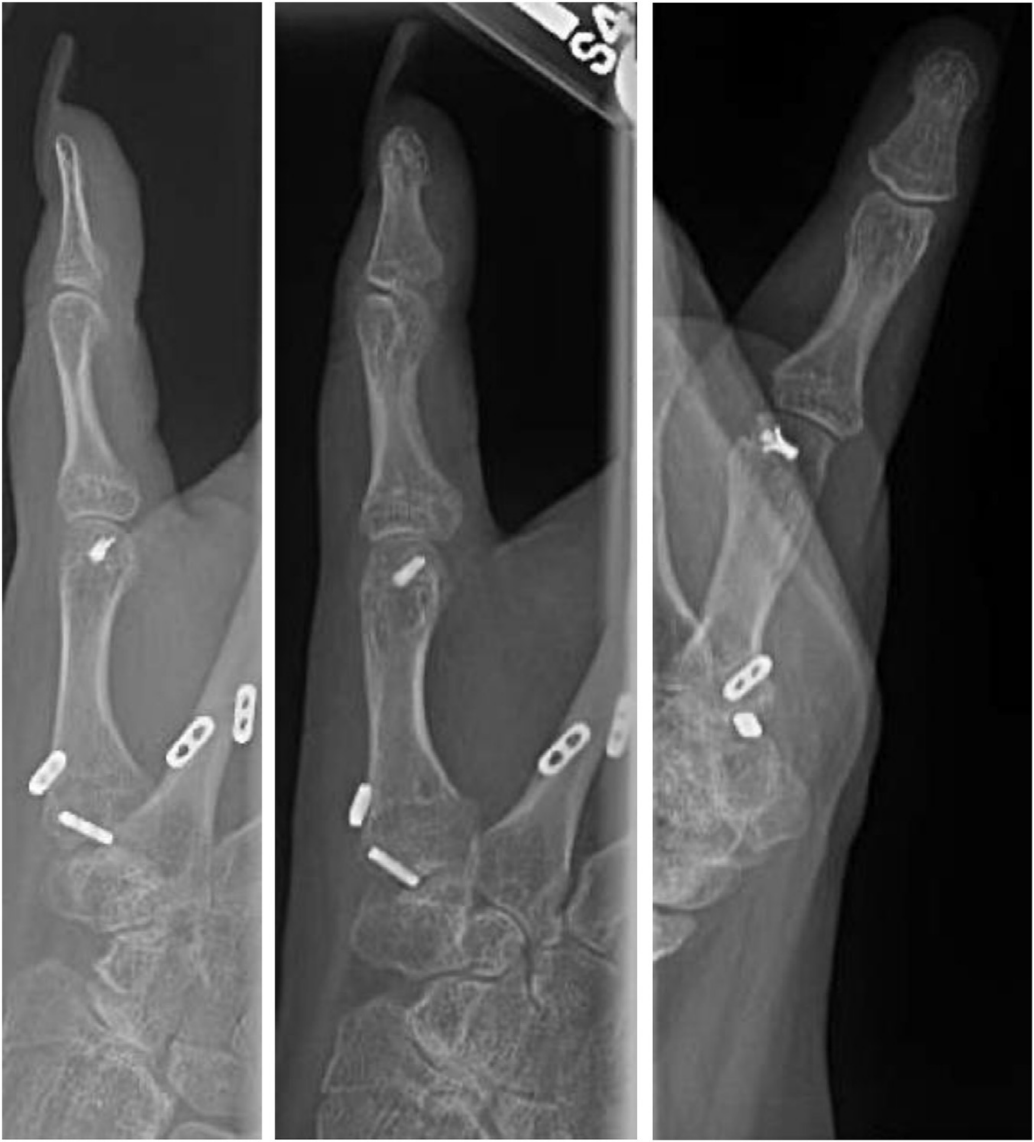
Two-year postoperative posteroanterior, oblique and lateral right thumb radiographs demonstrating stable alignment of the thumb CMC joint with interval reconstruction of the UCL of the MCP joint.
